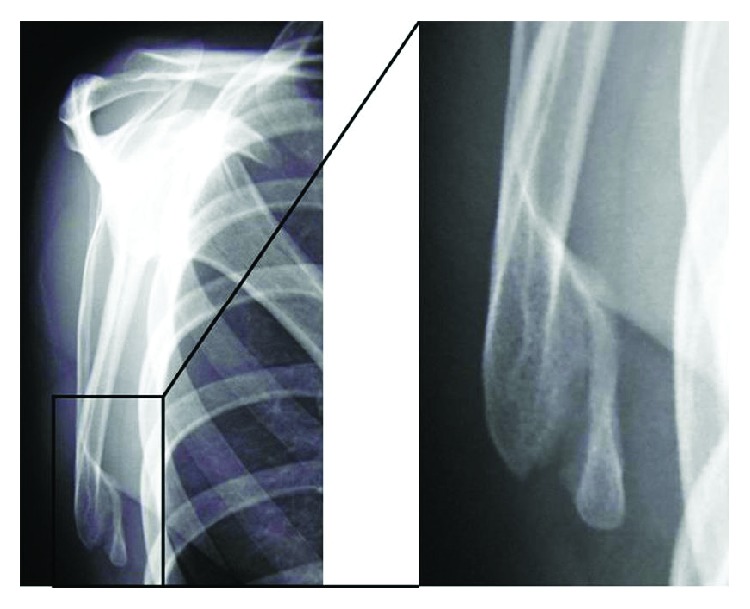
Radiographs showing a small bony fragment on the ventral side of the inferior scapular angle with a narrow space between the fragment and the scapular body to which the superior border was connected by a callus bridge.
